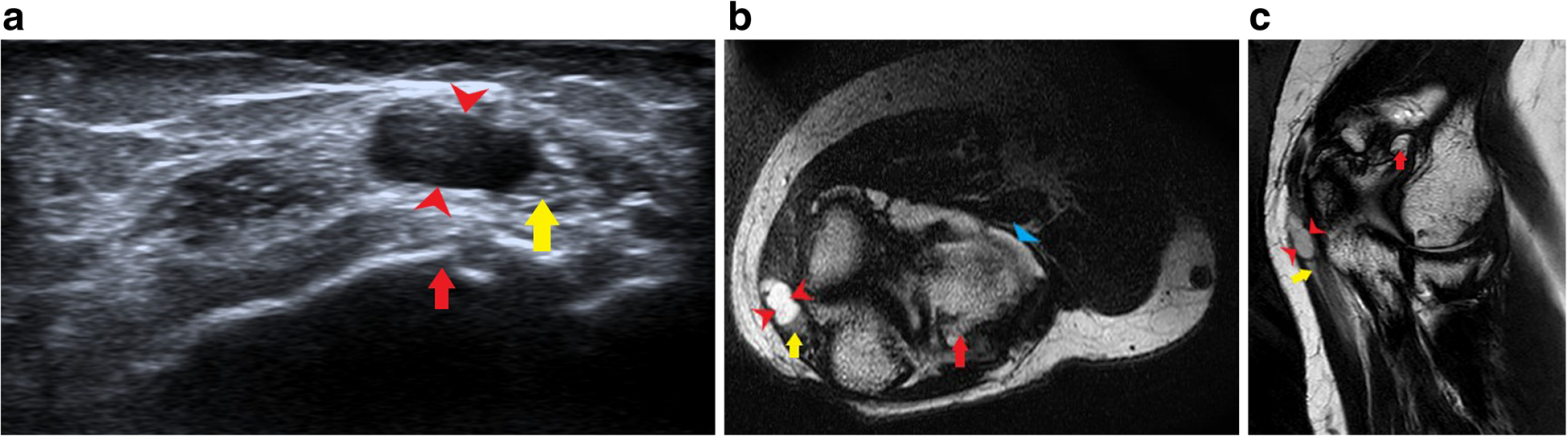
a Anechoic cystic lesion (red arrowheads) adjacent to the ulnar nerve (yellow arrow) at the elbow with underlying cortical erosions (red arrow). The cystic lesion appears to arise from the nerve, suggestive of an intraneural ganglion cyst. b T2-weighted axial section shows hyperintense cystic lesion (red arrowheads) which post operatively was diagnosed as ganglion cyst arising from the nerve sheath of the ulnar nerve (yellow arrow). The nerve is hyperintense due to compressive neuropathy. Also noted are osteophytes (red arrow) and joint effusion (blue arrowhead) suggestive of arthritic changes. c T2-weighted coronal image shows the cystic lesion (red arrowheads) arising from the adjacent ulnar nerve (yellow arrow) lying along the course of the nerve [54]. Also noted are osteophytes (red arrow)
